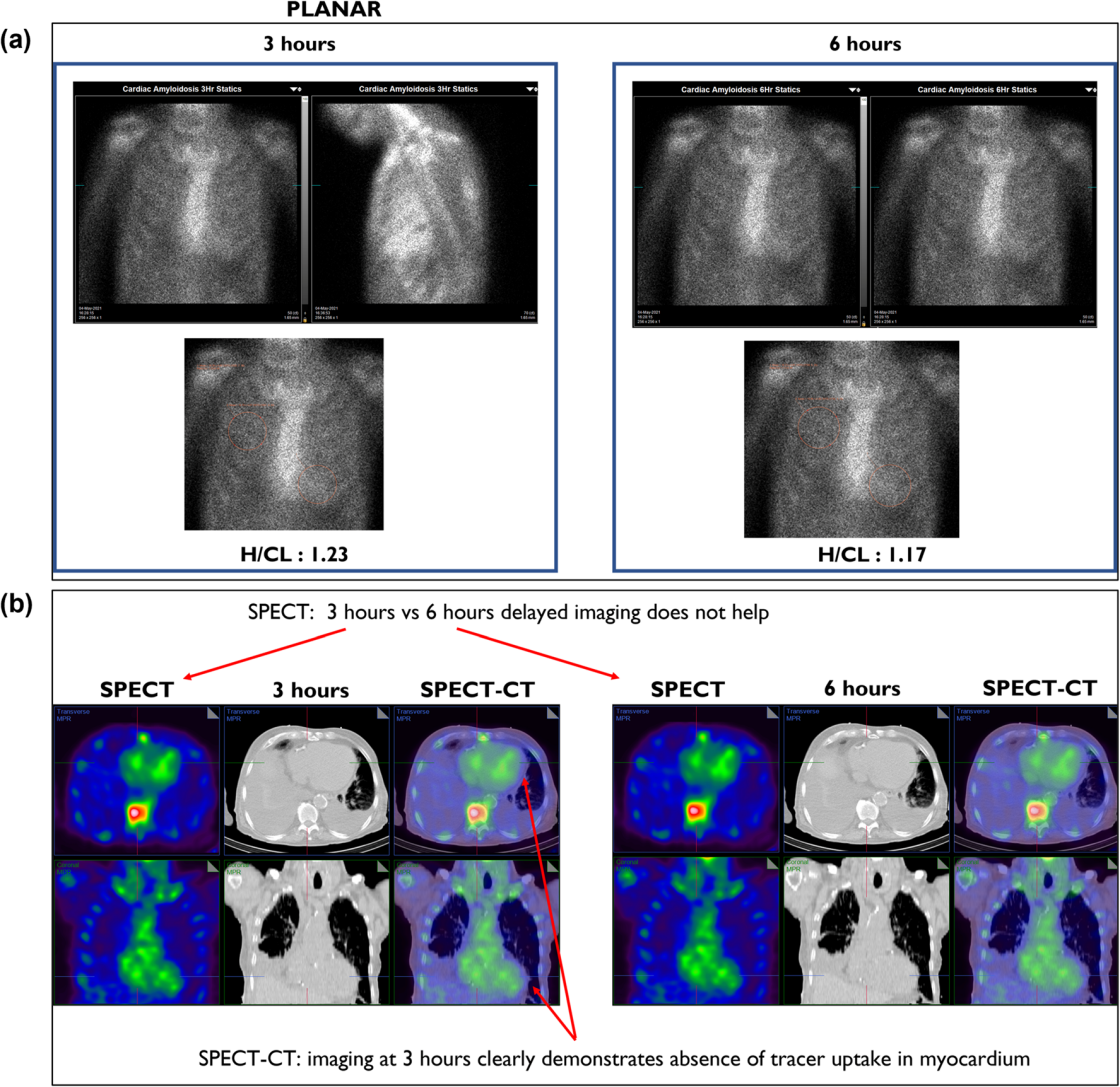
(A) Three-hours planar imaging *vs* six-hour planar imaging: H/CL ratio of 1.23 and 1.17 respectively, Grade 1 uptake in both planar images, demonstrating that 6 h delayed imaging does not add value. B SPECT/CT at three hours demonstrates absence of myocardial PYP uptake. SPECT/CT reclassifies case from equivocal to not suggestive
